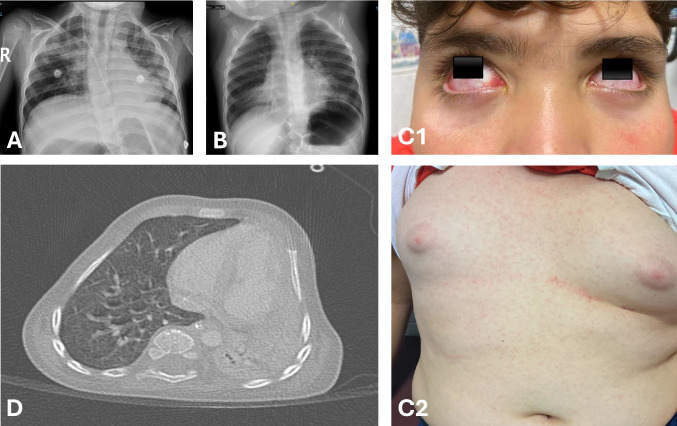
Multisystem involvement in a pediatric patient with adenovirus infection. A Chest X-ray demonstrating perihilar and basal infiltrates consistent with pneumonia. B Chest X-ray shows diffuse pulmonary opacities consistent with pneumonia. C1 Bilateral conjunctival injection. C2 Maculopapular rash on the trunk of the same patient as in C1, who was hospitalized and evaluated for multisystem inflammatory syndrome in children (MIS-C) in the differential diagnosis. D Thoracic computed tomography image demonstrating the right lung extending posterior to the sternum toward the left hemithorax, with marked volume loss and complete atelectasis of the lung
